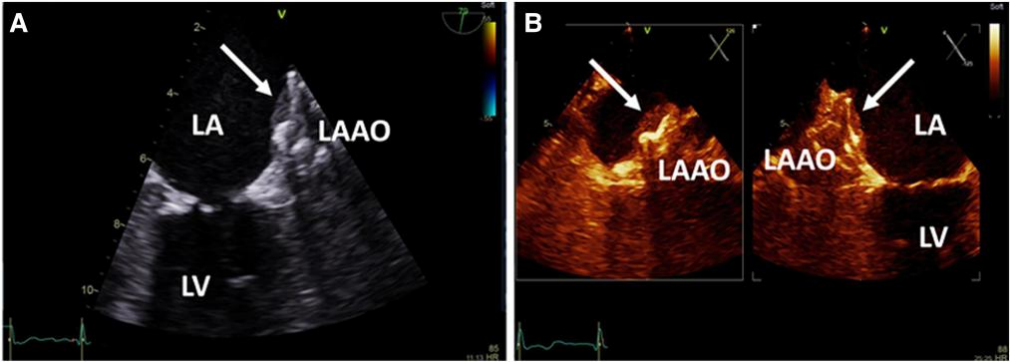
Follow-up transoesophageal echocardiography (TOE) 6 weeks after left atrial appendage occlusion (LAAO) procedure. *(A*) TOE demonstrating an echo-dense mass attached to the LAAO. *(B*) B-mode transforms the image from standard shades of grey to an alternative colour display and was used for better visualization of the mass on the LAAO. LA, left atrium; LV, left ventricle.
